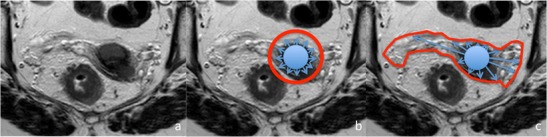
Axial high resolution T2-weighted image of the pelvis. a Fifty-six-year-old woman with synchronous malignancies of the rectum and cervix; b Centrifugal local tumour spread, irrespective of tissue boundaries, forms the basis of traditional cancer surgery, and dictates tumour excision with metrically defined margins; c According to the ontogenetic compartment theory of local tumour spread, tumour dissemination is not centrifugal, but rather confined to embryologically determined ontogenetic compartments until relatively late-stages. Resection along compartment borders is expected to lead to better disease control
